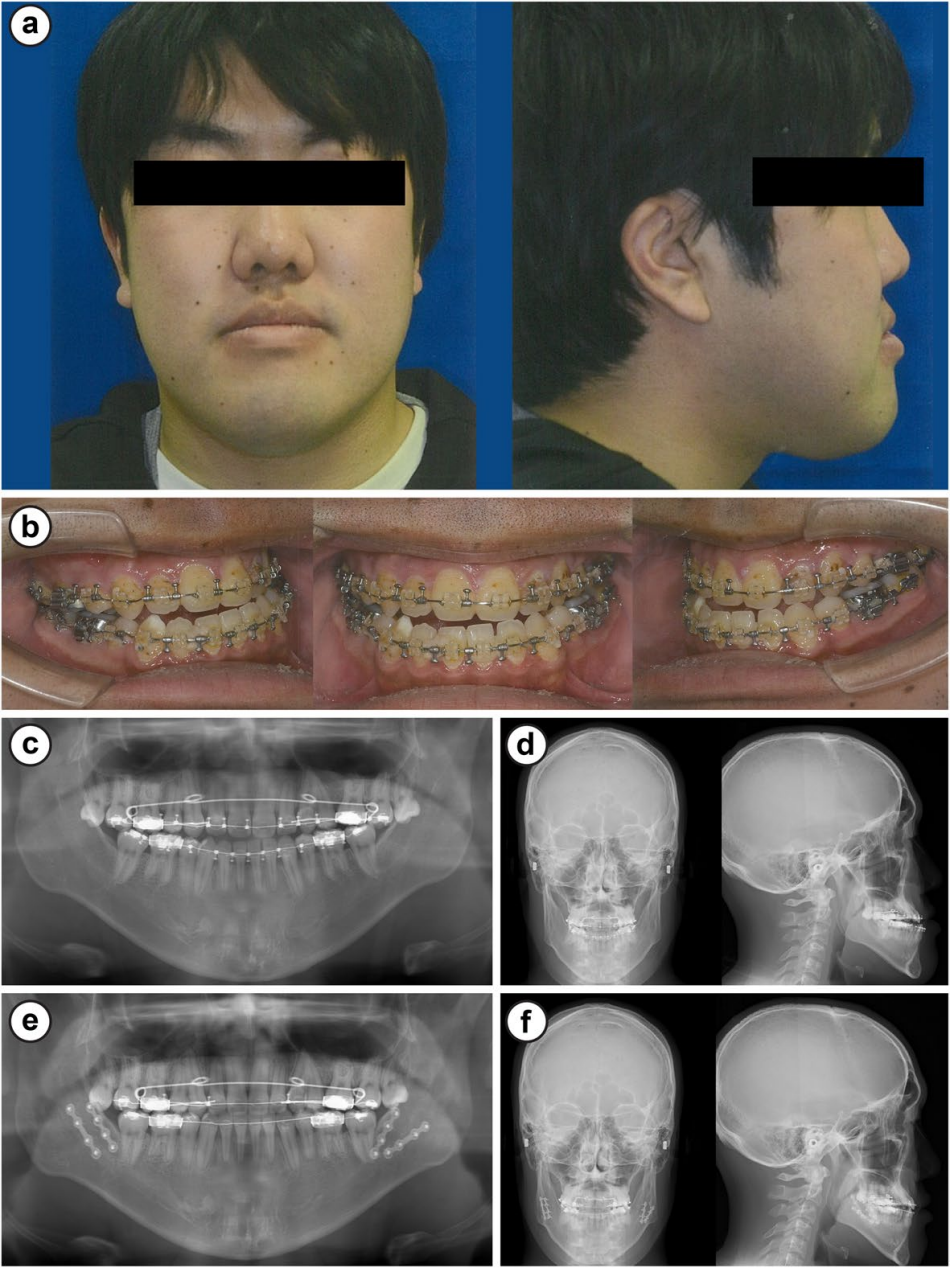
Case 1. a Facial photographs, b intraoral images, c panoramic radiographs, d cephalometric radiogram, e panoramic radiographs 1 month postoperatively, and f cephalometric radiogram 1 month postoperatively
